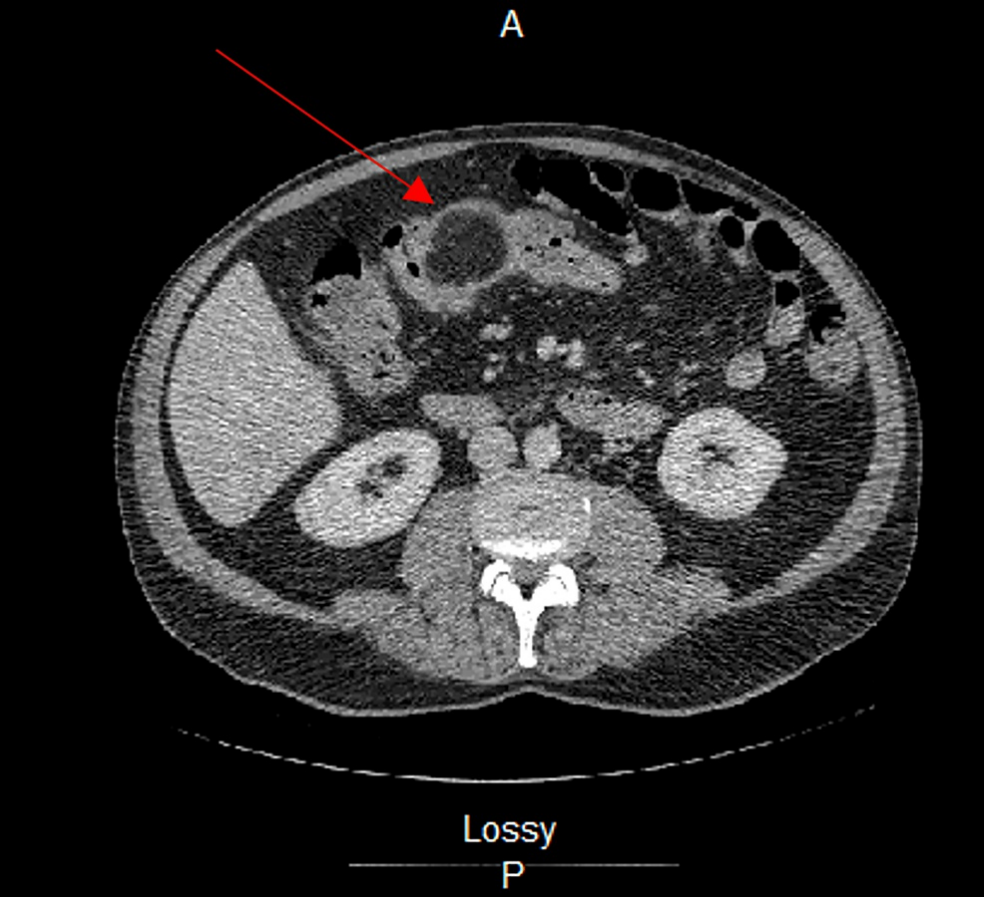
CT imaging with IV contrast revealed a benign lipoma located between the ascending and transverse colon. The lipoma presented as a well-circumscribed, thinly-encapsulated ovoid mass with low attenuation in the center.
